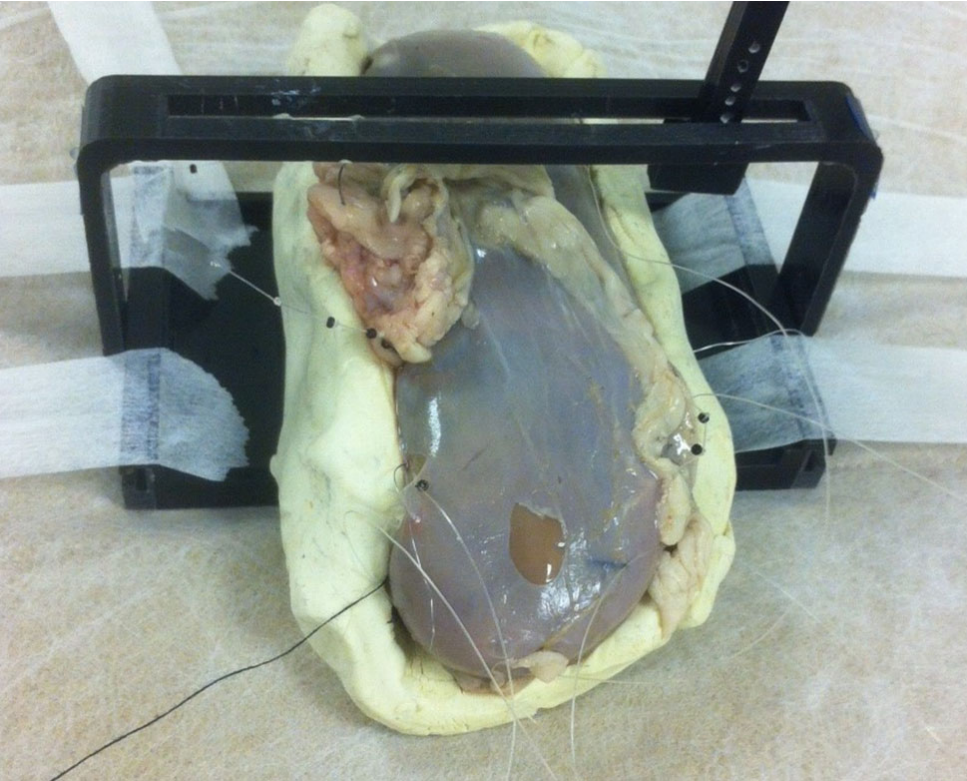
Deformation rig with kidney and support, placed on the CT scanner table
